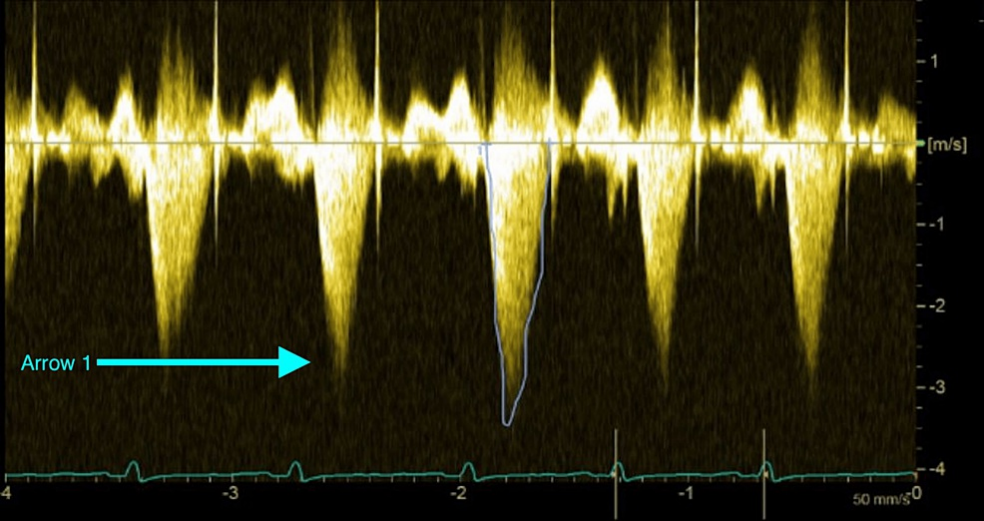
TEE image of continuous wave Doppler through the LVOT while the IABP was at 1:1 counterpulsation. This waveform demonstrates the characteristic “dagger shape” morphology of left ventricular outflow tract obstruction. Arrow 1 highlights the late systolic peak. TEE: transesophageal echocardiography; LVOT: left ventricular outflow tract; IABP: intra-aortic balloon pump
